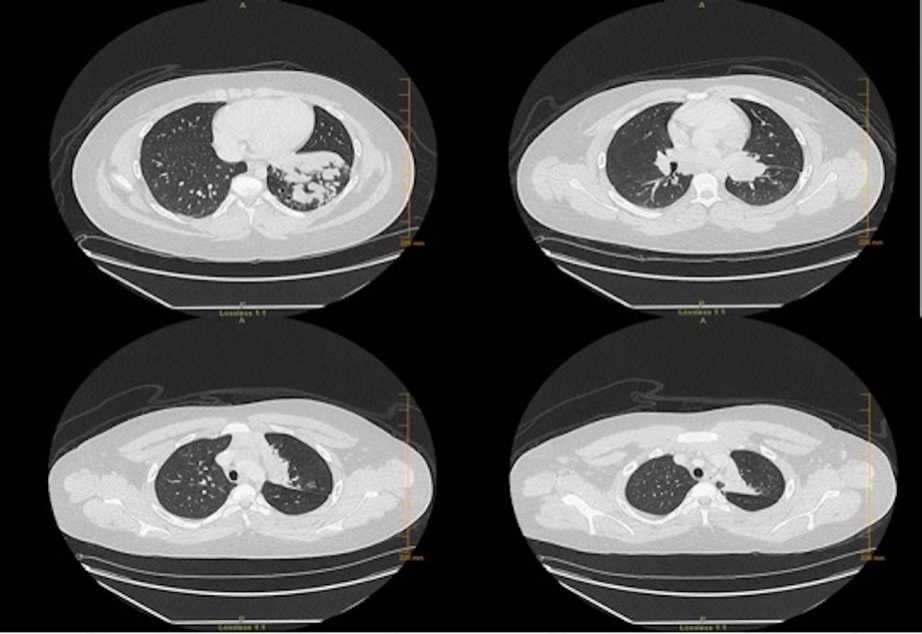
CT Scan: Complete occlusion of left main lower lobe bronchus extending to segmental and sub‐segmental bronchi with partial sparing of superior segmental bronchus, with opacification of diffusely dilated left lower lobe bronchi
